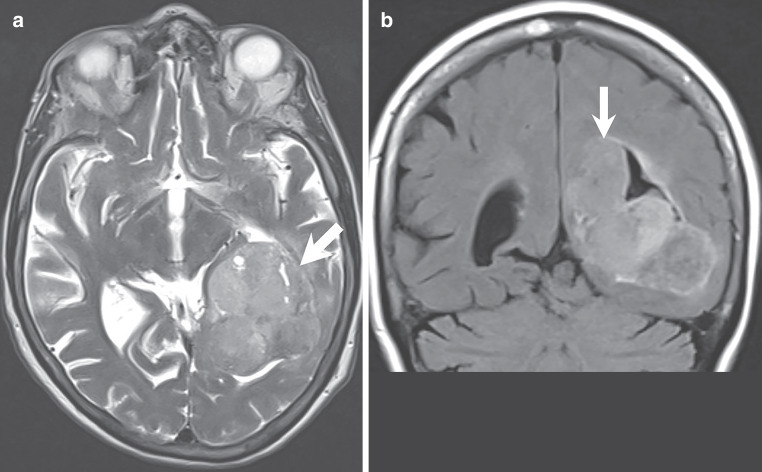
Axial T2-weighted images (a) and coronal FLAIR (Fluid Attenuated Inversion Recovery) images (b) reveal an intraventricular mass lesion (a, b, arrows) extending from the trigone (a) into the left lateral ventricle (b). The tumour matrix exhibits heterogeneous signal intensities on both T2-weighted and FLAIR images
